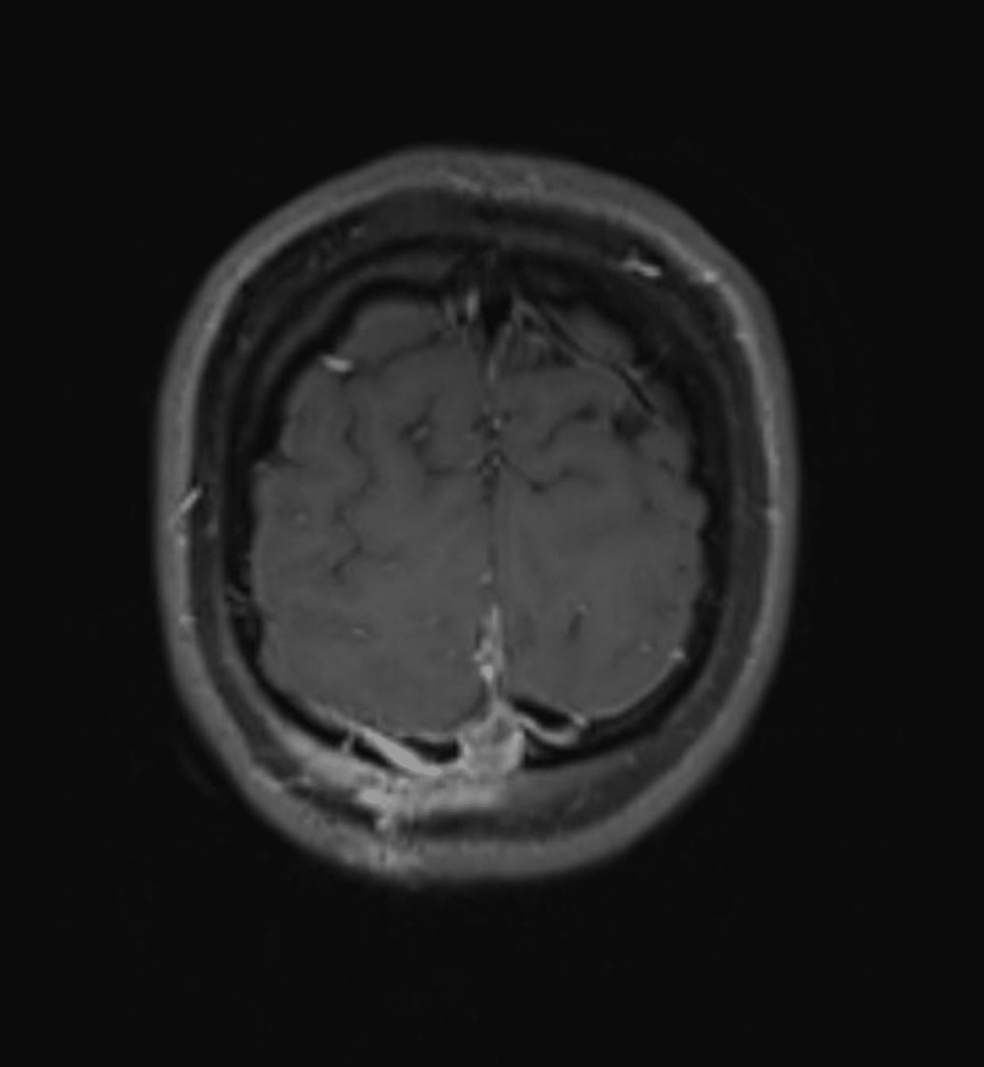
Postoperative magnetic resonance imaging scan in the coronal view. The scan does not show any residual disease.
